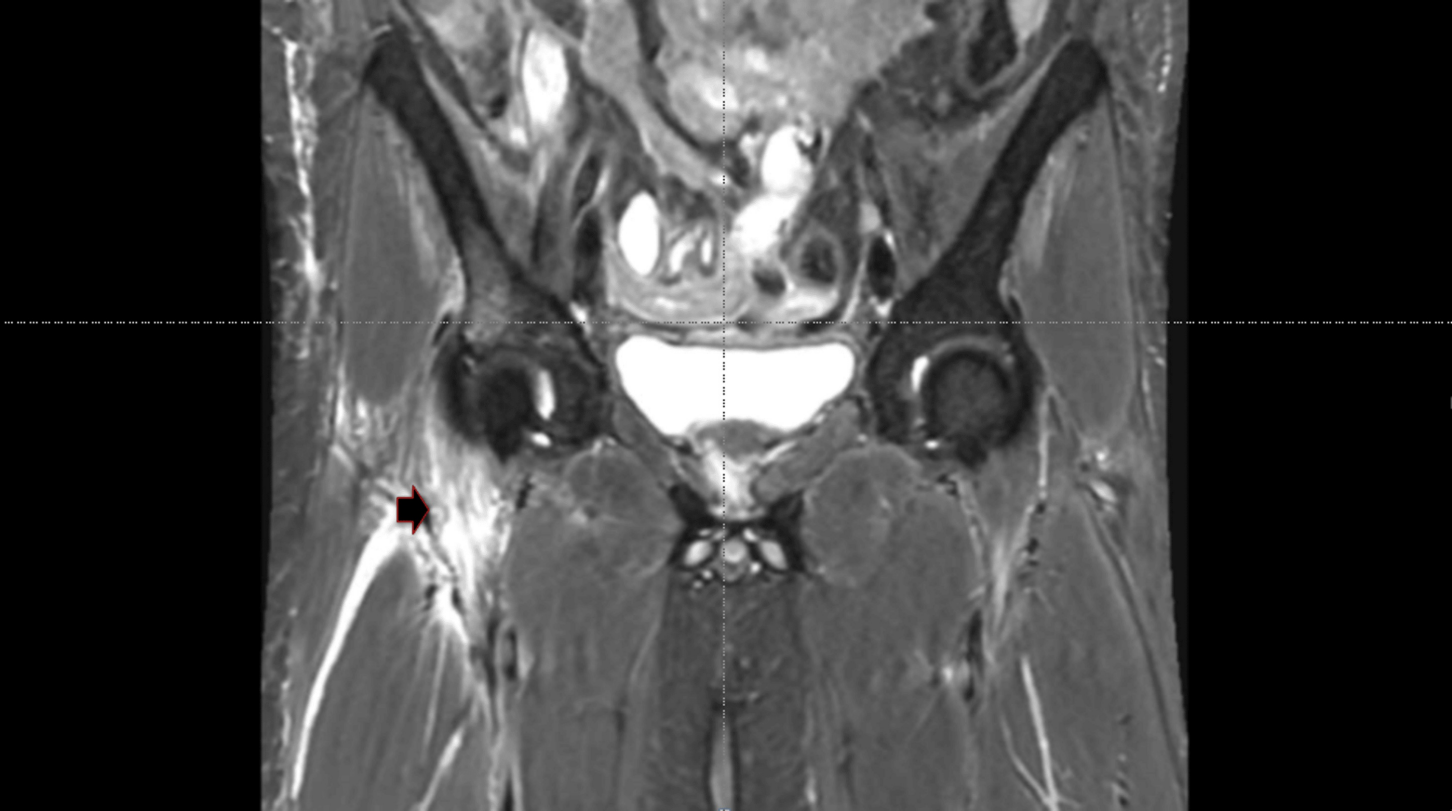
Magnetic resonance imaging of the pelvis showing a right anterior superior iliac spine abscess
